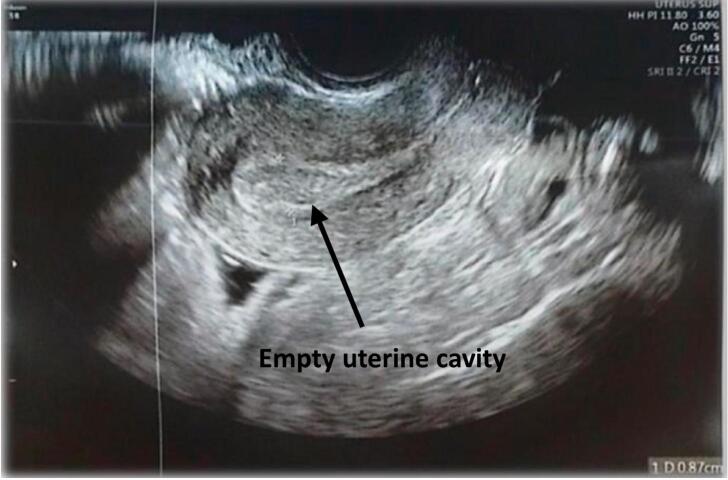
Transvaginal ultrasound showing an empty uterine cavity.
